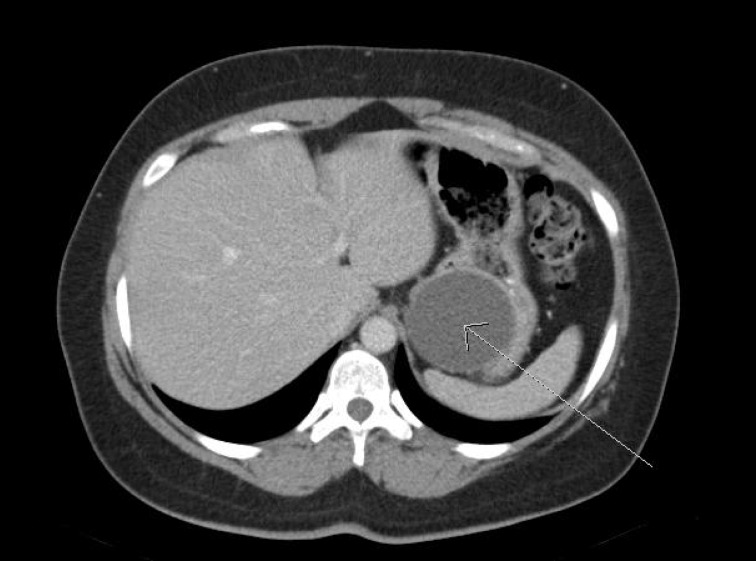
Contrast enhanced computed tomography of a gastric duplication cyst (arrow)
